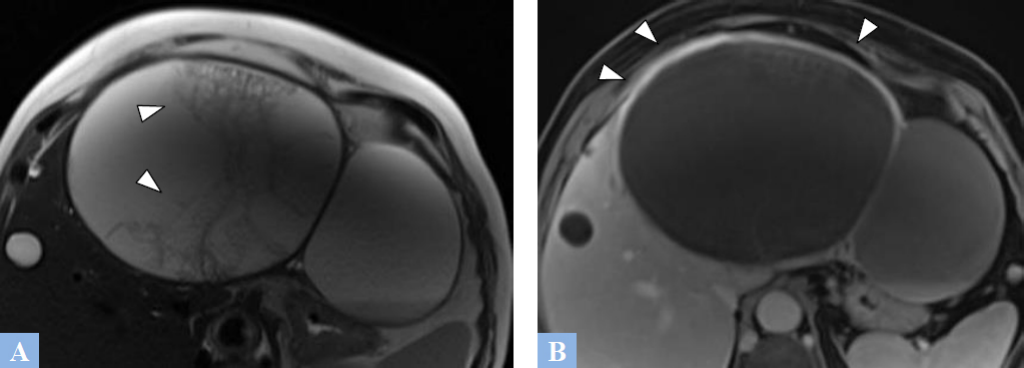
Infected biliary cyst in a 63-year-old female patient presenting with febrile pain in the right hypochondrium. MRI features. A. T2-weighted MR image in the axial plane. Cystic lesion in segment IV with a thick wall and heterogeneous content (arrowhead). B. T1-weighted fat-suppressed MR Image in the axial plane after gadolinium administration at the portal phase of enhancement. Contrast enhancement of the cyst wall is consistent with cyst infection (arrowheads).
